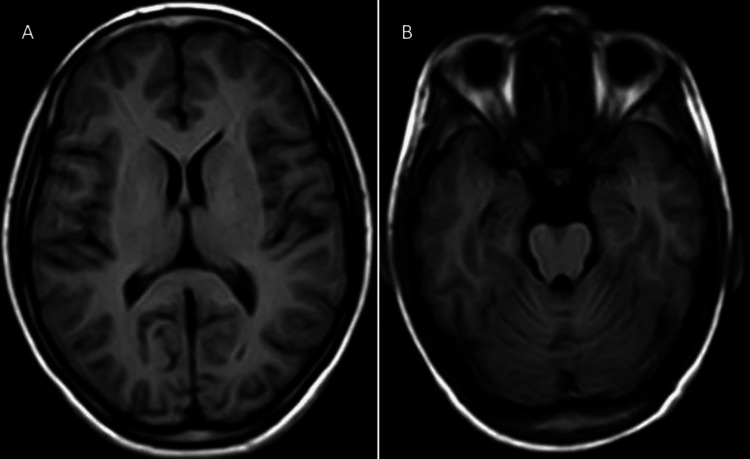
Normal MRI brain of the patient (A, B) MRI: Magnetic Resonance Imaging
